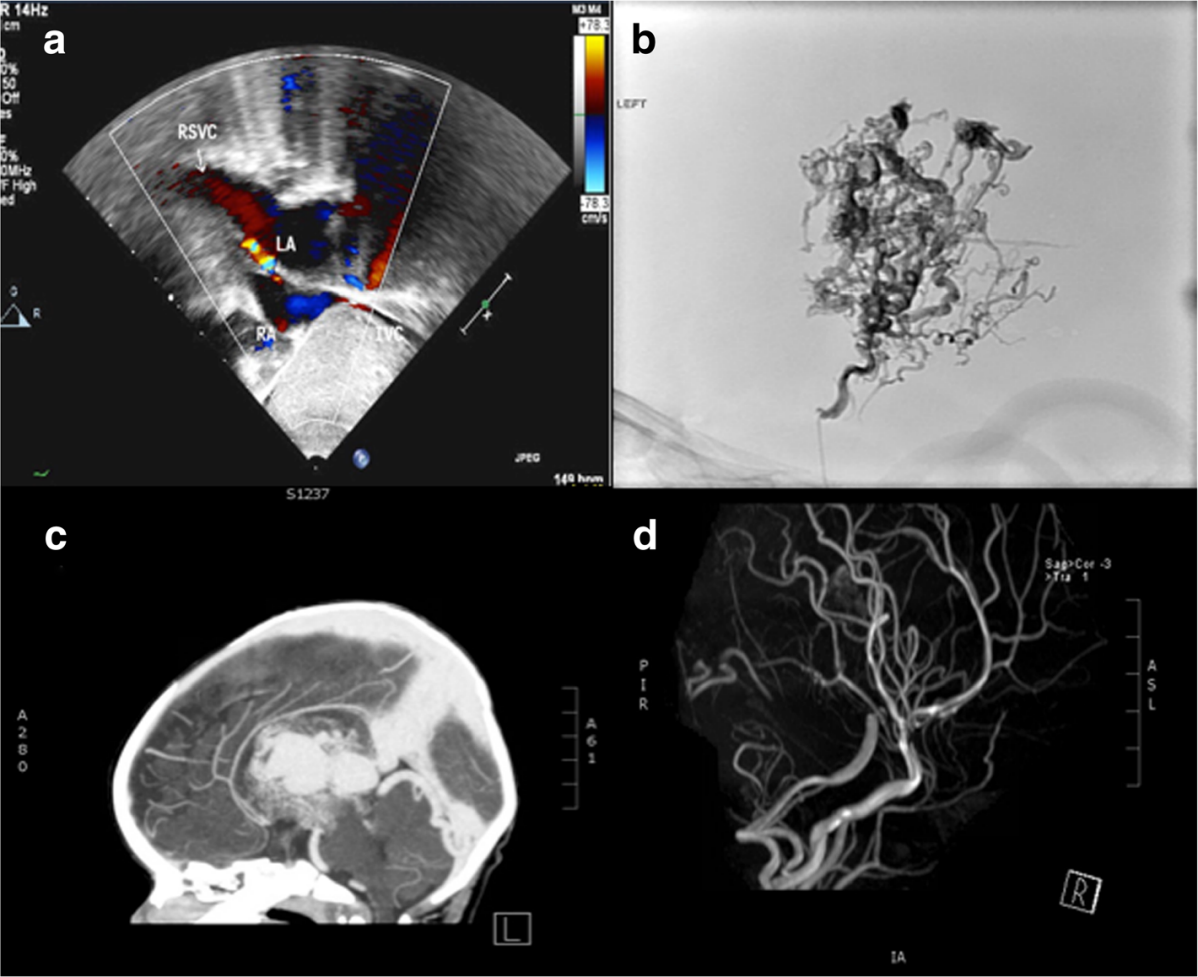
a Echocardiography modified bicaval view showing dilated right superior vena cava (RSVC) draining into dilated the dilated left atrium (LA). Inferior vena cava (IVC) is draining normally to the right atrium (RA). b Selective Angiography with multiple supplying vessels of a large arteriovenous malformation deeply located in the left basal ganglia with drainage into MProsV of Markowski that drains into the vein of Galen. c Moderate hydrocephalus is present. The maximum size of the arteriovenous malformation is approximately 4.9 cm in size. The Arterial supply from middle cerebral artery and anterior cerebral artery small branches. The venous drainage through MProsV of Markowski that drains into the vein of Galen with significant dilatation of the torcula and the transverse sinuses. d MR angiography and MR venogram: Complete obliteration of the arteriovenous malformation and the arteriovenous shunting. No further embolization is needed
